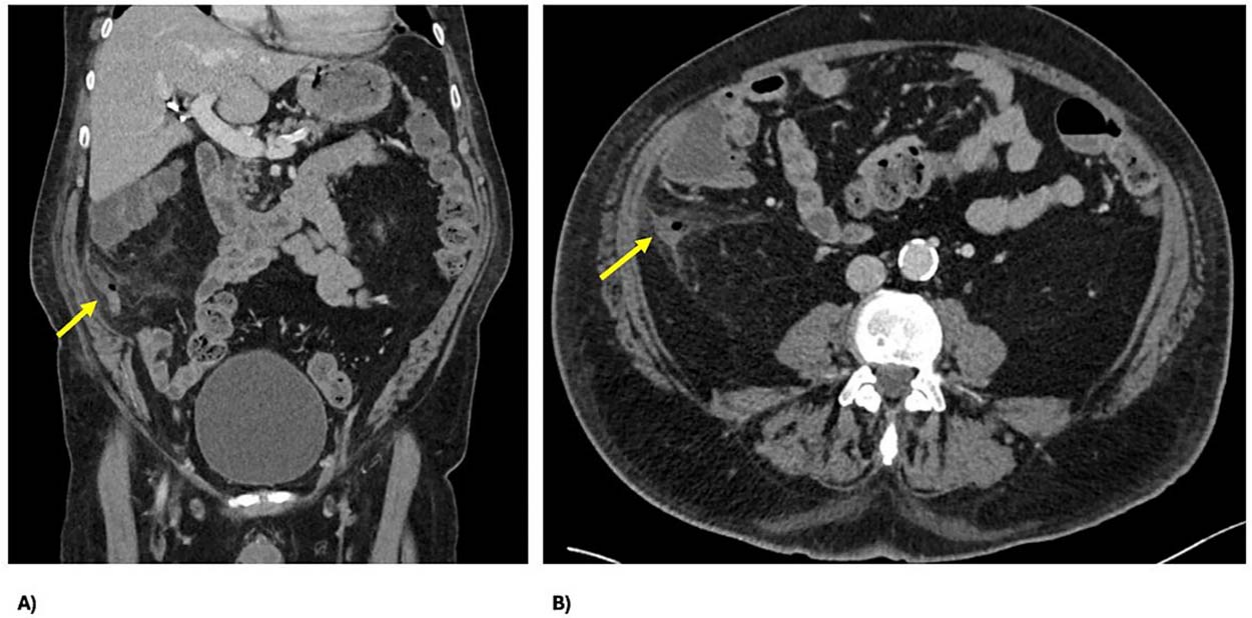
CT demonstrating perforated appendicitis post-embolization with a fluid collection and stranding, with the line of demarcation demonstrating hypoperfusion, suggestive of ischemia; (A) axial view and (B) coronal view.
